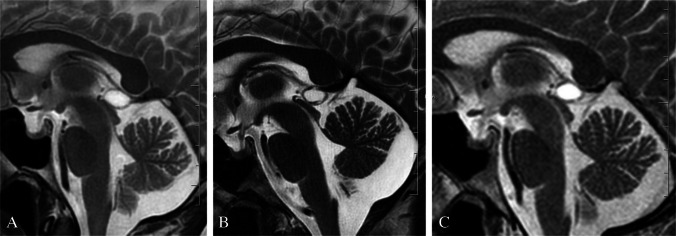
MRI of triplets with pineal cysts. A Sagittal T2-weigthed image of Triplet 1. B Sagittal T2-weigthed image of Triplet 2. C Sagittal T2-weigthed image of Triplet 3
